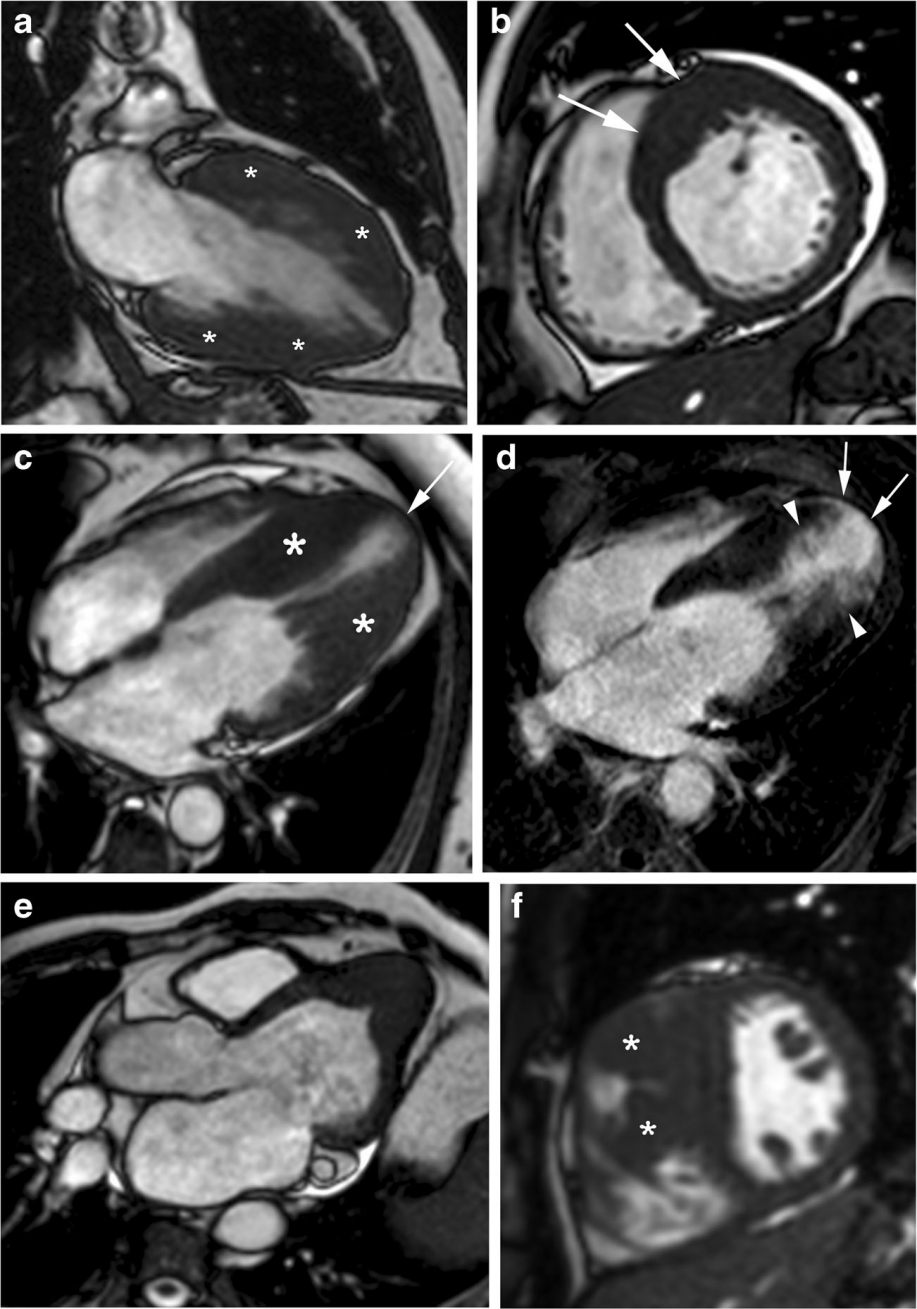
Distribution of myocardial hypertrophy in classic HCM phenotype. a, 56-year-old man with symmetric myocardial hypertrophy. End-diastolic two-chamber SSFP cine MR image shows diffuse and symmetric LV wall hypertrophy (asterisks); b, 43-year-old man who presented with palpitations. Short-axis SSFP cine MR image at end-diastole shows asymmetric septal wall hypertrophy (arrows); c, 57-year-old man with severe dyspnea. End-diastolic four-chamber SSFP cine MR image demonstrates a “dumbbell” configuration of the LV cavity with midventricular myocardial thickening (asterisks), marked muscular midcavity systolic constriction and a thin-walled apical aneurysm (arrow). d, Four-chamber LGE MR image clearly depicts the enhanced thinned apical LV aneurysm (arrows) extending from the aneurysm rim into the septum and free wall (arrowheads). Global systolic function also decreased because the ejection fraction was 35%; e, 53-year-old woman with apical HCM. End-diastolic horizontal long-axis SSFP MR image demosntrates the spade-like appearance of LV chamber secondary to apical LV hypertrophy; f, 22-year-old man with right ventricular hypertrophy. Short-axis SSFP cine MR image at end-diastole shows a prominent right ventricular structure that inserts directly on the anterior ventricular septum corresponding to large hypertophied crista supraventicularis muscle (arrowheads)
